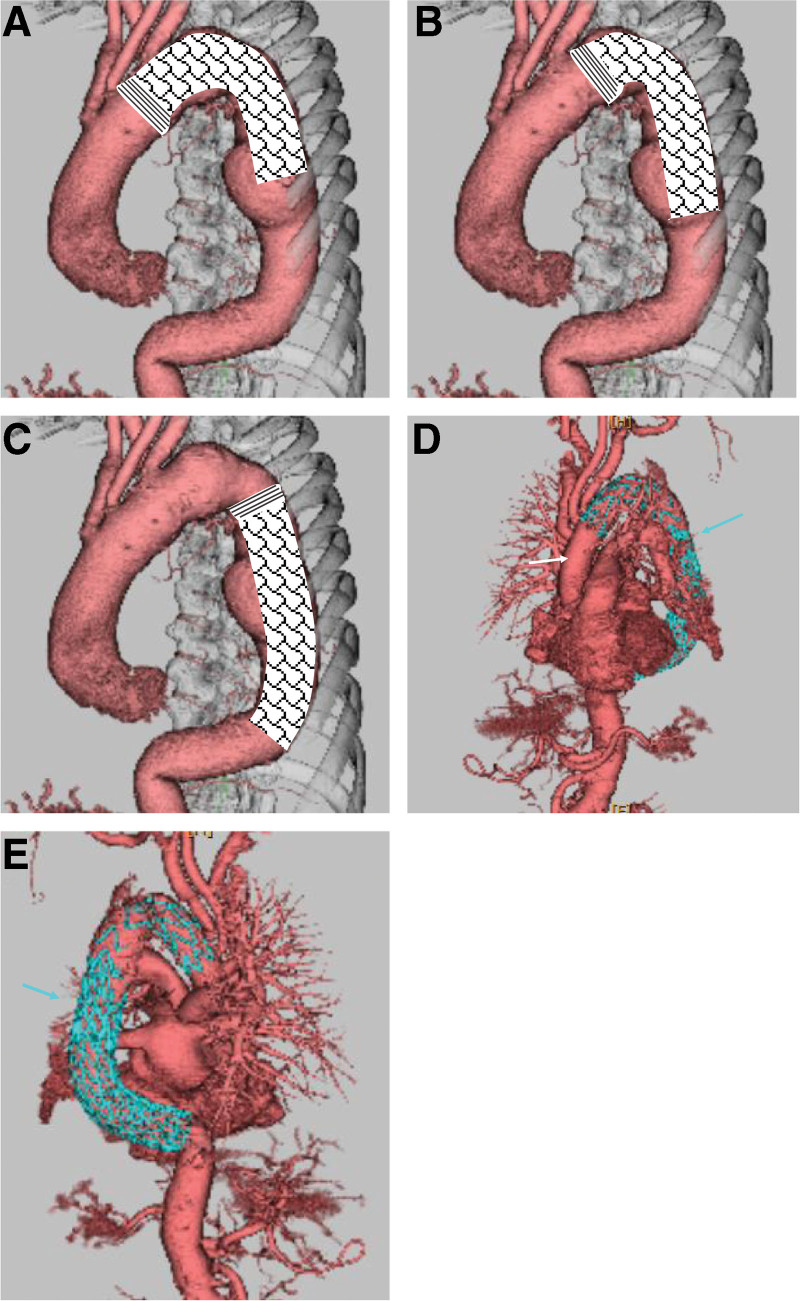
Three-dimensional computed tomography for surgical prediction. (A) Predicted CT with distal anastomosis in zone 2 and insertion of 150 mm of the FET, where the FET does not cover the descending aortic aneurysm. (B) Predicted CT with distal anastomosis in zone 3 and insertion of 150 mm of the FET, where the FET does not cover the descending aortic aneurysm. (C) Predicted CT of a distal aortic arch aneurysm in zone 3 with distal anastomosis on the distal side and insertion of 150 mm of the FET. The FET covers the descending aortic aneurysm but may exceed the Adamkiewicz artery. (A–E) Postoperative 3-dimensional contrast-enhanced CT. The white and blue arrows indicate the graft for total arch replacement and the stent graft for the descending aortic aneurysm, respectively. CT = computed tomography, FET = frozen elephant trunk.
